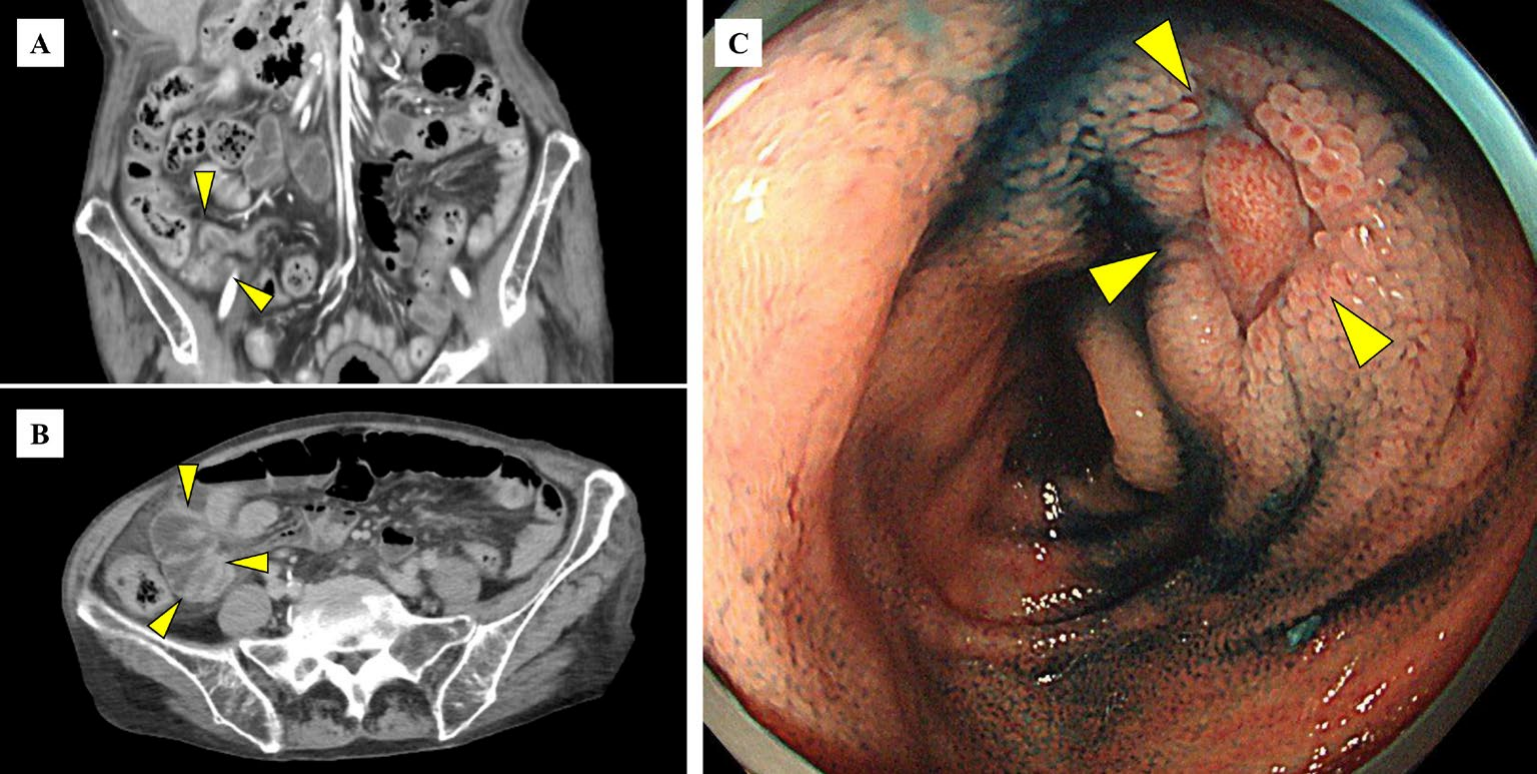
Finding of image. (A, B) Contrast‐enhanced computed tomography (CT). (C) Colonoscopy. (A, B) Abdominal contrast‐enhanced CT shows enteritis predominantly involving the terminal ileum with a contrast enhancing mesentery (yellow arrowheads). (C) Colonoscopy shows an ulcer (yellow arrowheads) located in the terminal ileum.
